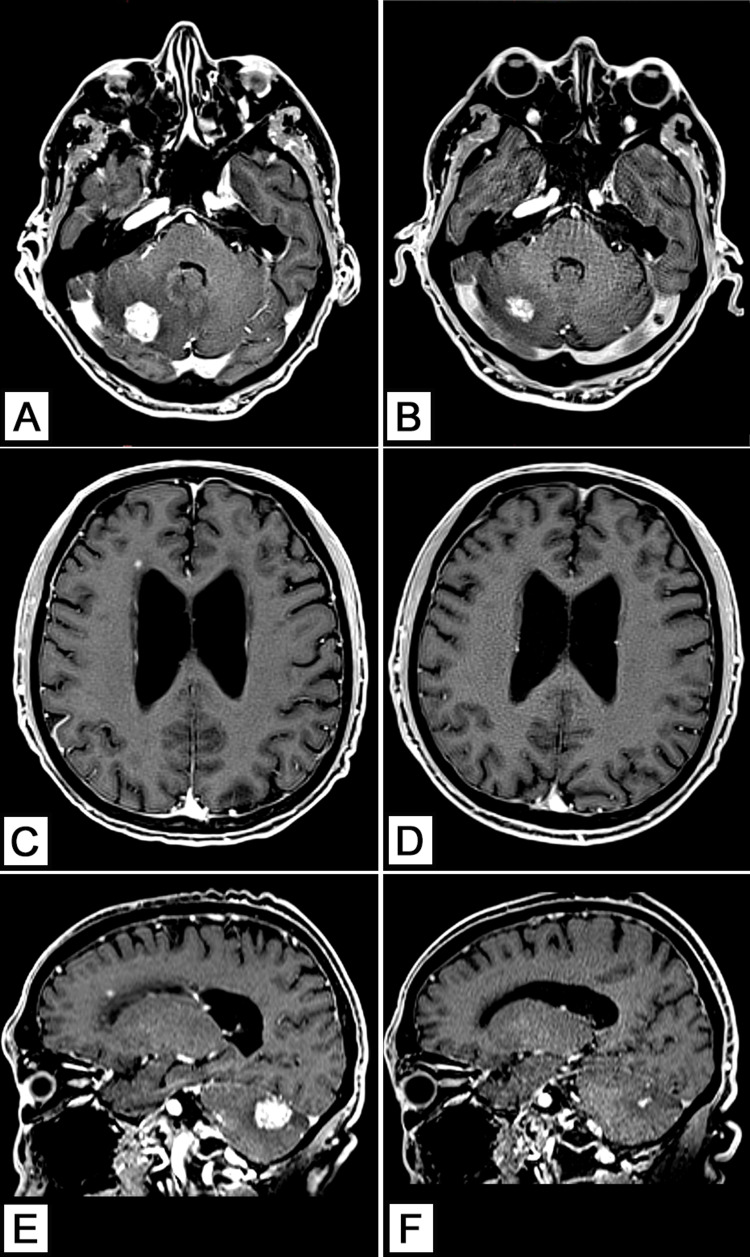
Pre- and post-treatment comparison scans (A, C, E) Pre-treatment contrast-enhanced MRIs. (B, D, F) Post-treatment scans showcasing a significant reduction in lesion size. (B) Post-treatment contrast-enhanced axial MRI shows a significant reduction of the lesion diameter and complete resolution of the mass effect affecting the fourth ventricle. (D) Post-treatment contrast-enhanced axial MRI reveals a complete resolution of the small metastatic lesion anterior to the frontal horn of the right lateral ventricle. (F) Post-treatment contrast-enhanced sagittal MRI clearly shows a remarkable reduction in the size of both lesions.
